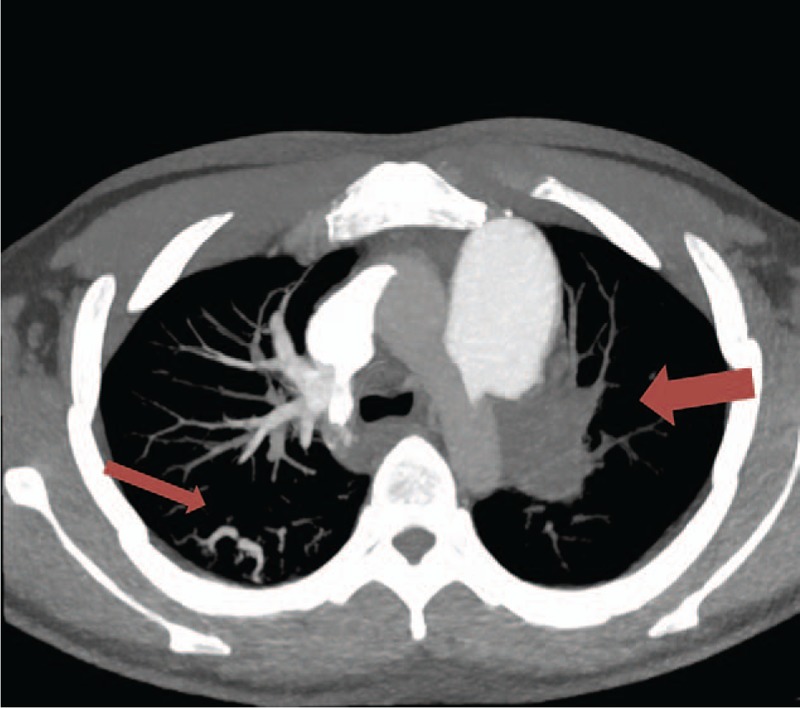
Computed tomography pulmonary angiography revealed multiple pulmonary embolisms, including a complete embolism of the left pulmonary artery (PA) and partial in the branches of the right PA.
